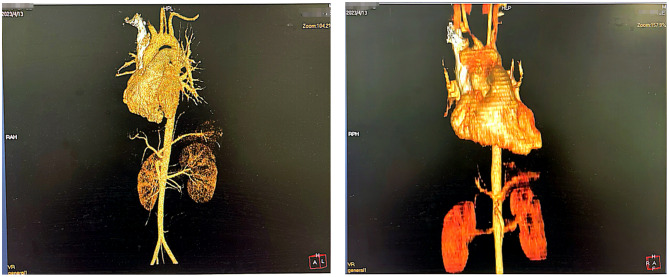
CTA of the chest and abdomen. No effusion shadow was seen in the pleural cavity on both sides. Filling defects of varying sizes were seen in the distal part of the original right pulmonary artery trunk and its branches, and in the left lower pulmonary artery and its branches, which had disappeared. All cardiac cavities were well filled with contrast medium, and a mass-like filling defect was still seen in the right ventricle, with a cross-sectional size of about 7 mm x 5 mm, slightly reduced from the previous one, and the atrial septum was not obviously defective, and the left vertebral artery originated from the aortic arch. The right diaphragmatic surface was slightly elevated
